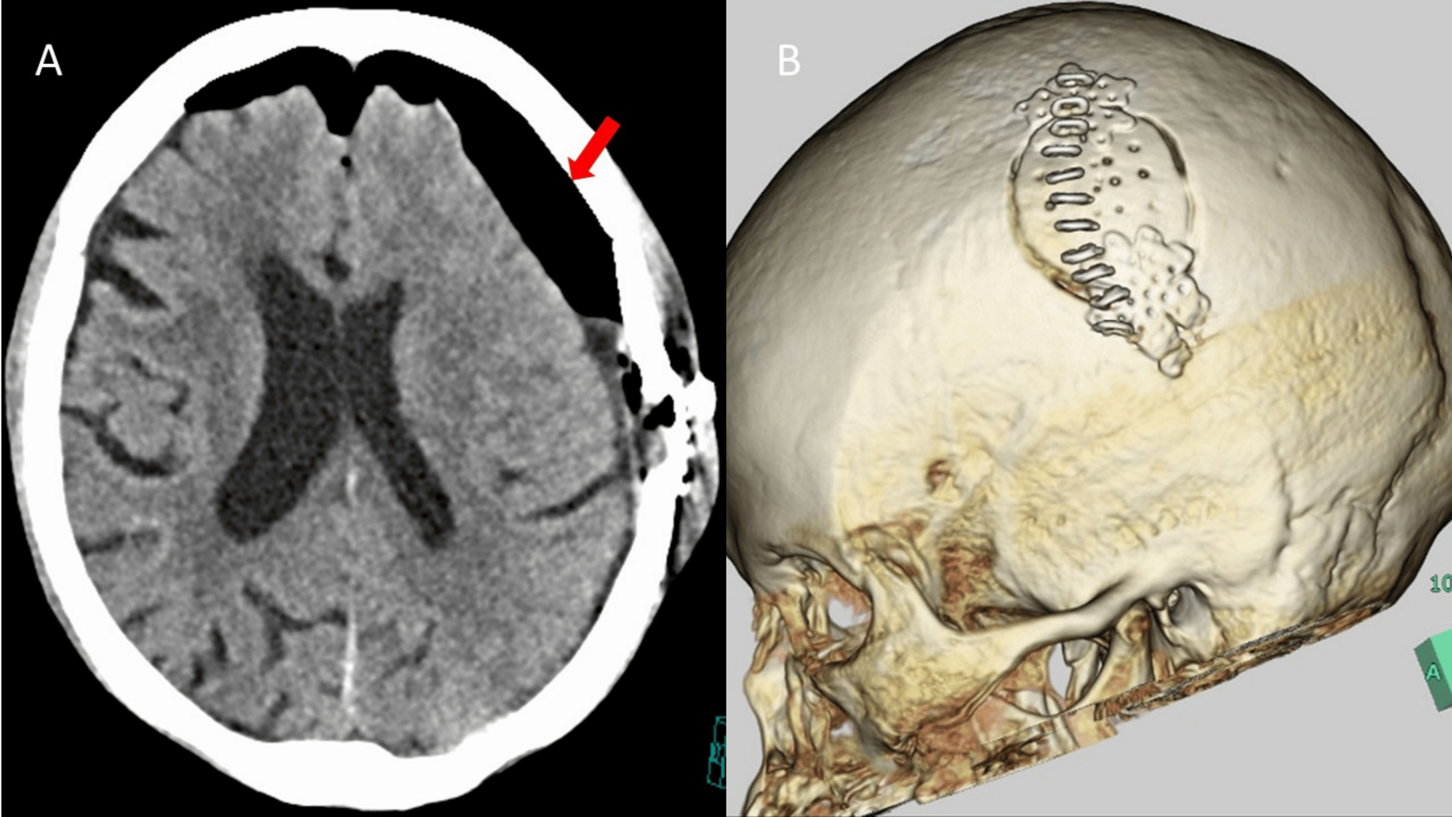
(A) Postoperative head CT obtained immediately after surgery showing complete removal of the hematoma (arrow) and improvement of mass effect. (B) Postoperative 3D CT image demonstrating the 7-cm linear skin incision and the craniotomy site corresponding to the thickest portion of the scalp (subcutaneous) hematoma.
